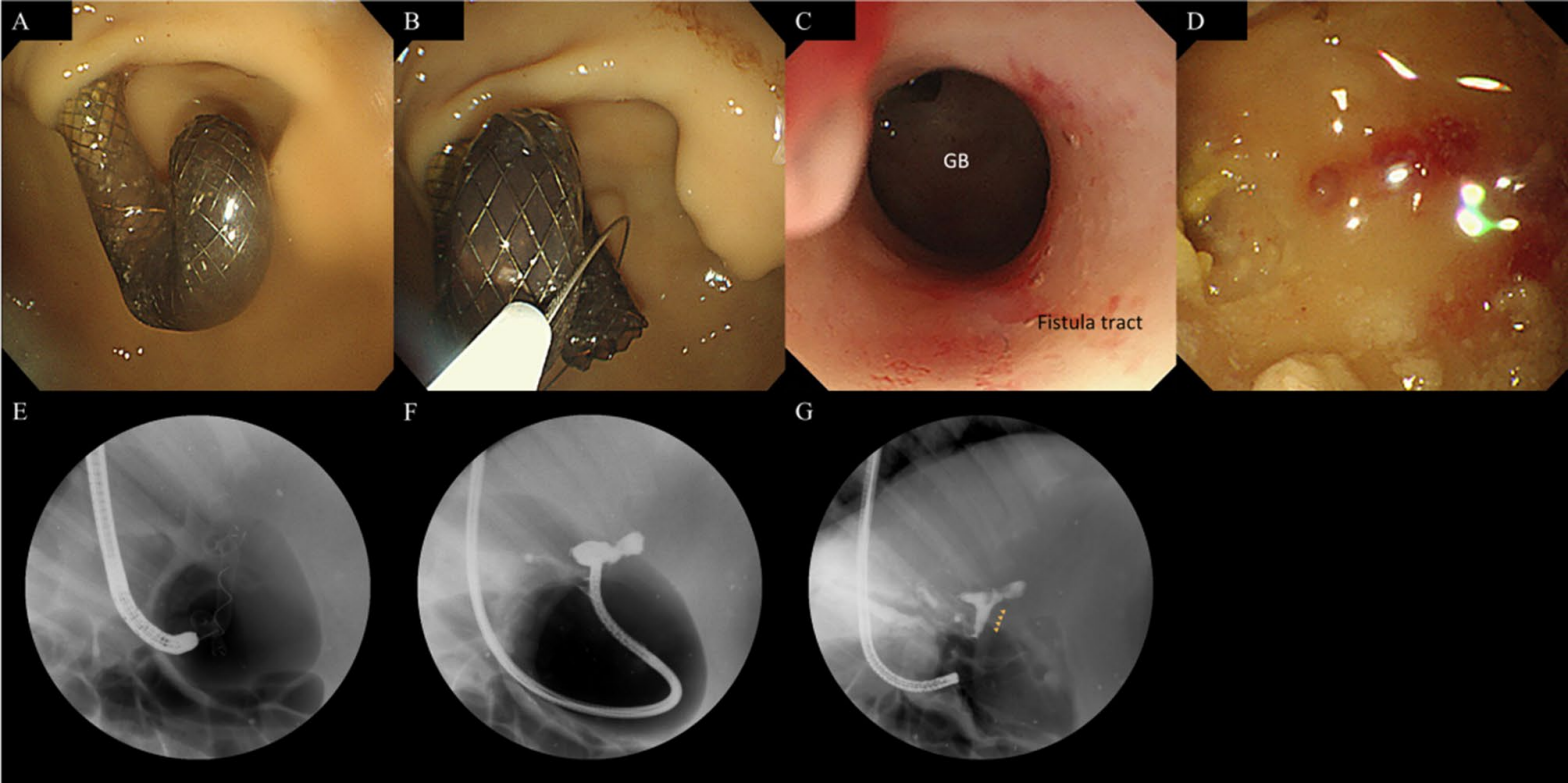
Follow-up endoscopy performed at 42 days after EUS-guided cholecysto-gastrostomy. (A, B) Endoscopic view showing removal of a stent by a snare. (C, D) Endoscopic view of lumen of gallbladder through the fistula tract by slim gastroscope. (E) Radiograph showing removal of a stent by a snare. (F, G) Contrast injection revealing a patent cholecysto-gastric fistula tract without any leakage (arrow: fistula tract).
